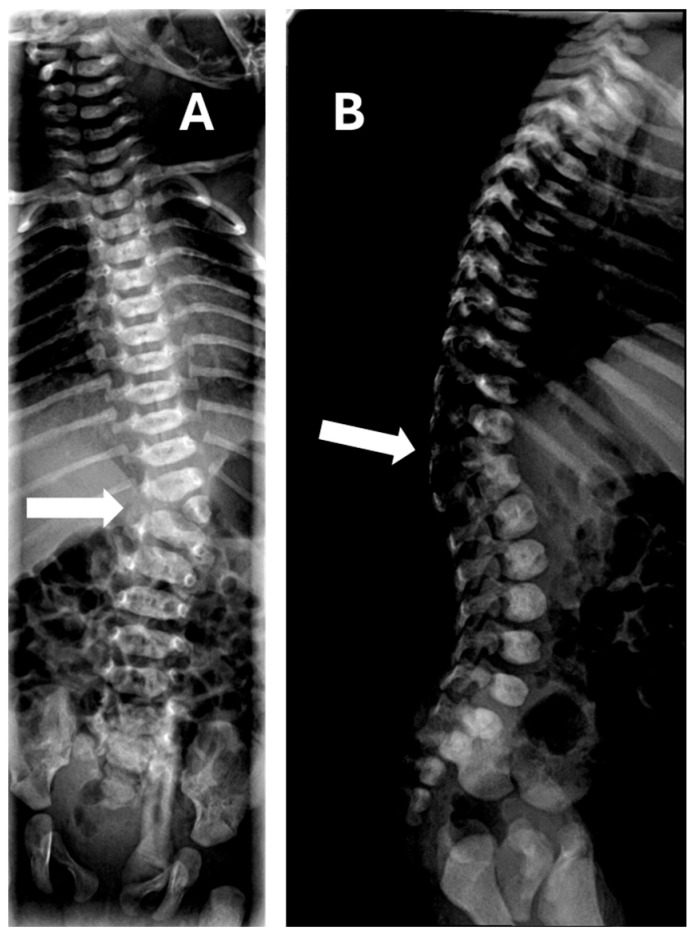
Postnatal radiograph of the spine at 3 months of age: (A) anteroposterior view and (B) lateral view, showing the presence of a lumbar hemivertebra diagnosed prenatally (arrows).
